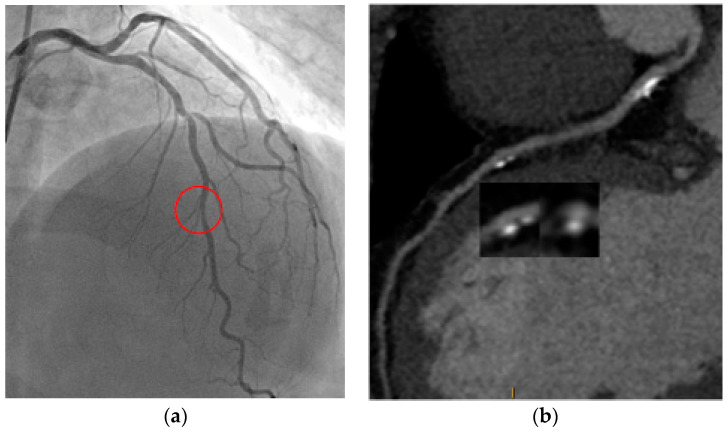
S.M. 54 years-old, male; dyslipidaemia, smoker, PAD; shortness of breath; CCTA (a): high risk plaque feature in left descending artery with spotty calcifications, positive remodeling; ICA (b): significant tandem-stenosis of LAD. PAD: peripheral artery disease; CCTA: coronary computed tomography angiography; ICA: invasive coronary angiography; LAD: left anterior descending artery.
